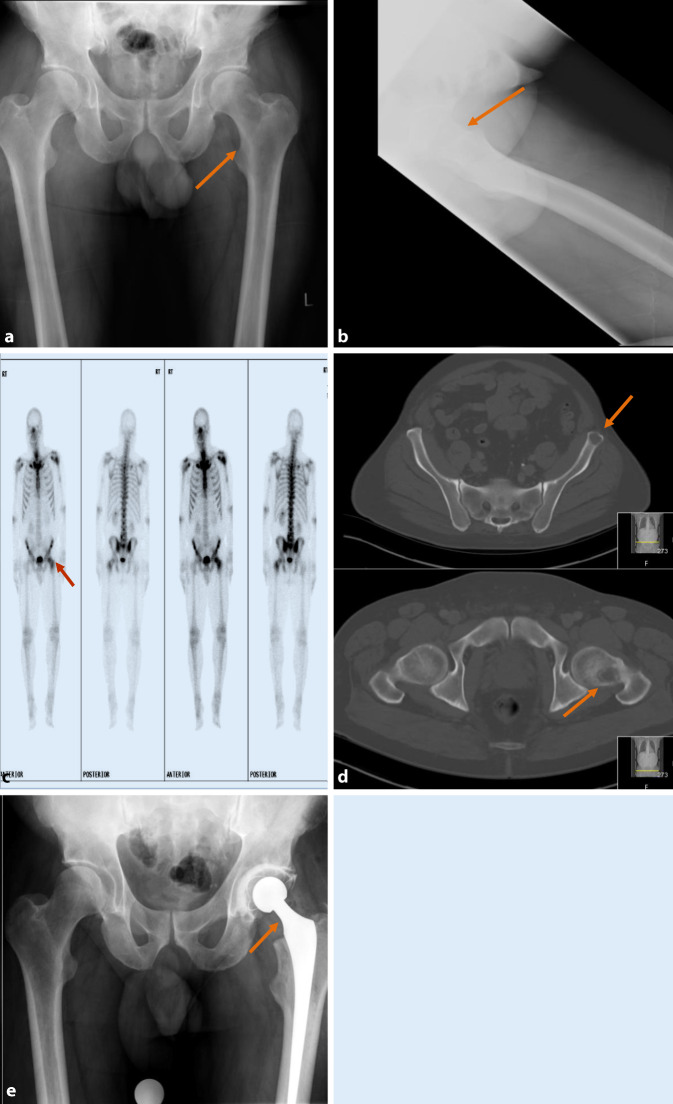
a, b Plain radiographs, c bone scan, d computed tomography (CT) scan bone windows, and e postoperative radiograph: A patient with a Mirels’ score of 6 for a lucent lesion in the left femur, confirmed on bone scan. The CT scan estimates the lesion to be larger than was seen on plain films and estimated at 2/3rd, raising Mirels’ score to 12. After a clinical consultation, it was established that the patient’s symptoms were constant, not just related to activity. The primary neoplasm was lung with a favorable prognosis. The patient was offered prophylactic total hip replacement with excellent postoperative recovery and remains in remission following cancer therapy
